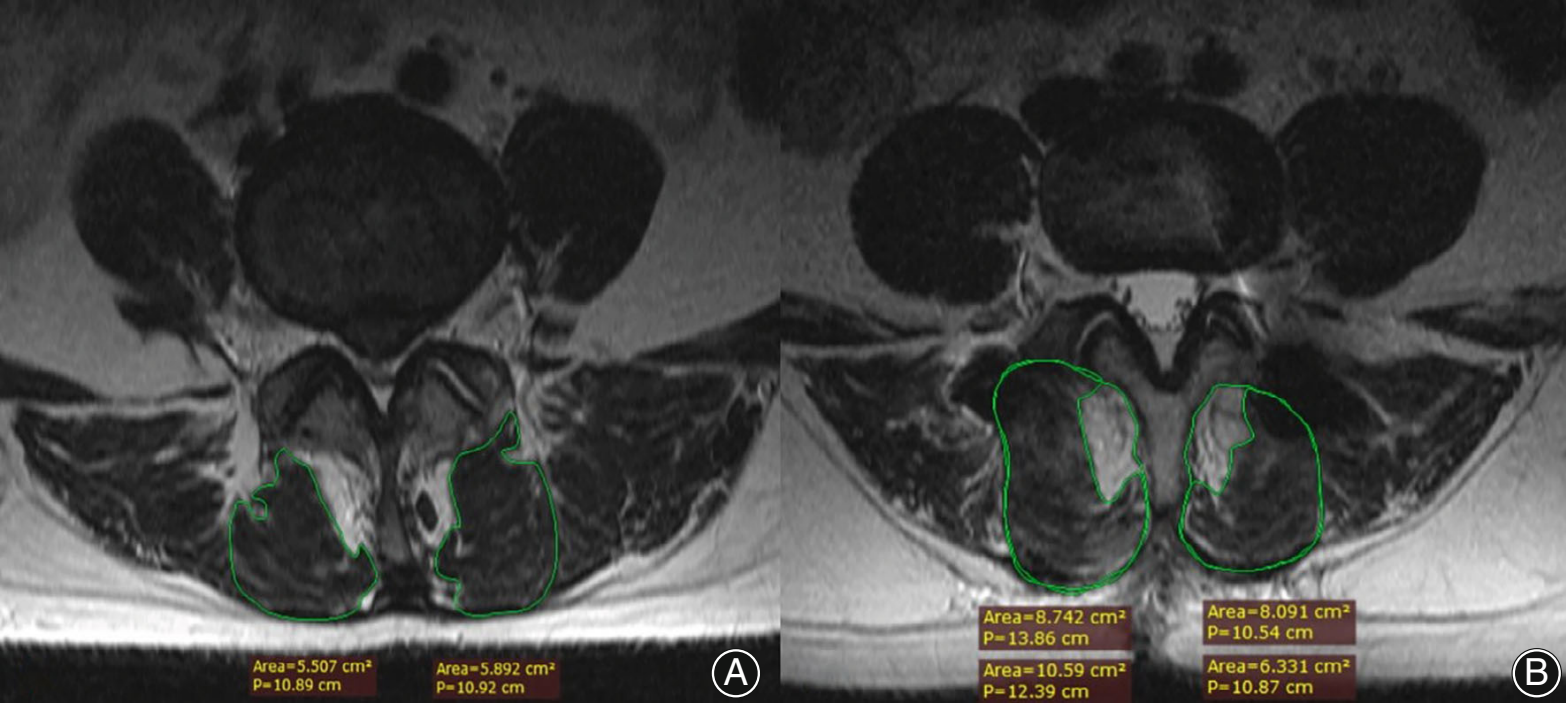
(A) Preoperative axial T2‐weighted MRI image demonstrates lean cross‐sectional area measurements. (B) Postoperative axial T2‐weighted MRI image demonstrates total and lean cross‐sectional measurements
